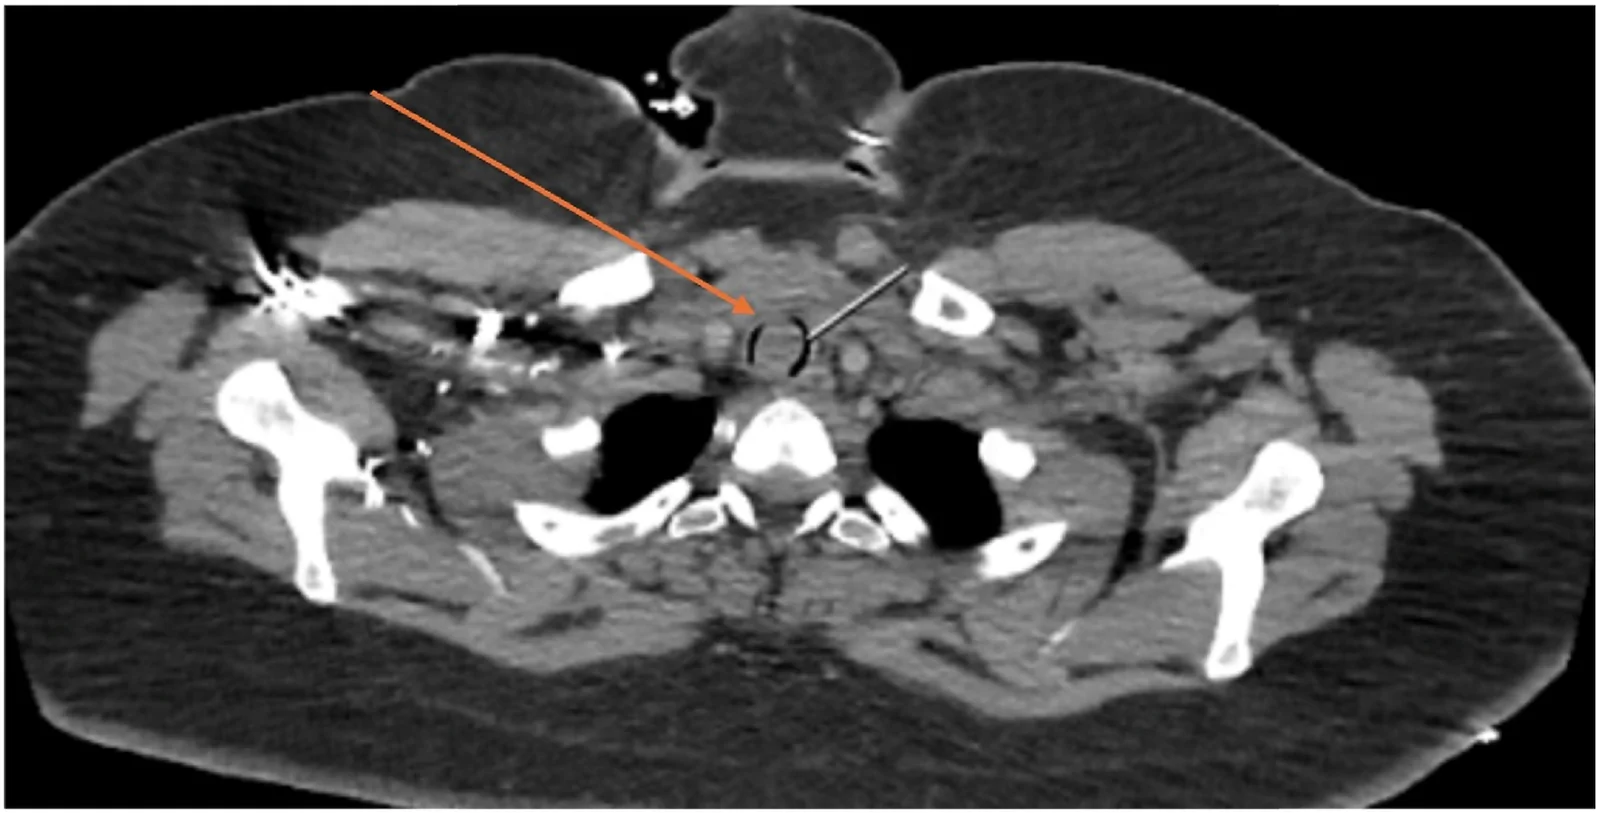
Axial chest computed tomography (CT) scan at the level of the thoracic inlet demonstrating a well-circumscribed, soft-tissue mass arising from the posterior membranous wall (arrow), resulting in critical, near-total mechanical obstruction of the tracheal lumen.
